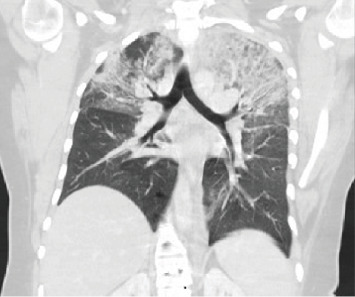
CT of the chest without contrast demonstrating bilateral upper lobe ground-glass opacities.
